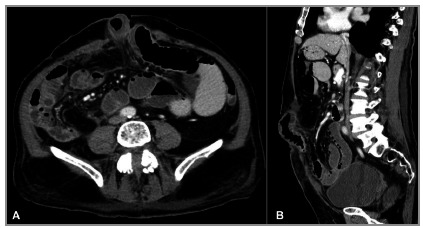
CT image showing a strangulated abdominal hernia: small bowel loop segment with signs of ischemia characterized by parietal thickening, mesentery densification, fluid in the hernia sac, and slight reduction in contrast uptake. In addition to distention of the proximal intestinal loops.
